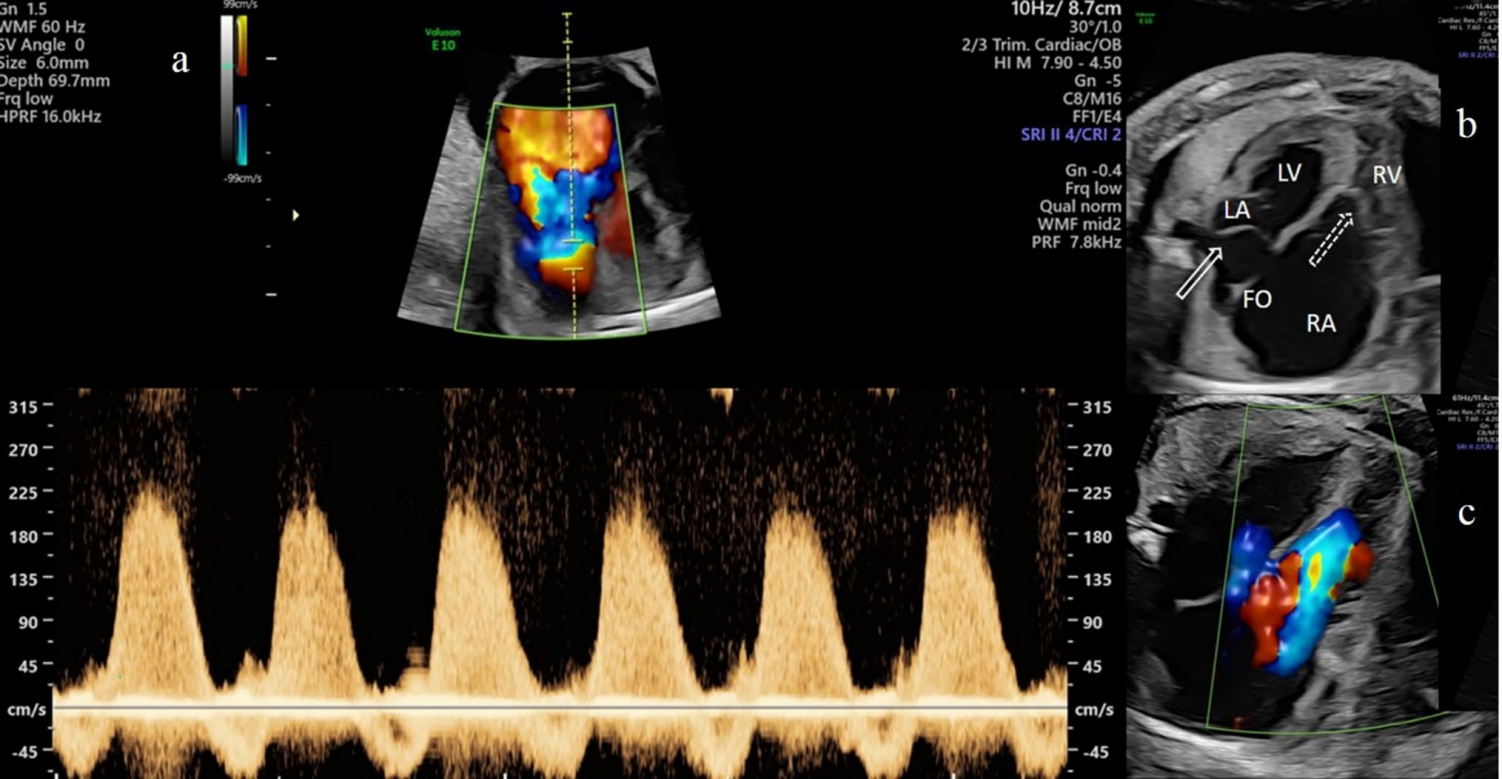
Case 1: a Holosystolic tricuspid regurgitation with peak velocity of nearly 230 cm/s shown in a fetus with severe Ebstein anomaly at 31 + 6 weeks of gestation. Color contours appears as a “hot air balloon”. b Apical four chamber view in grey scale demonstrates the severe cardiomegaly with the typical apical displacement of the tricuspid valve (open dotted arrow), with a FO (foramen ovale) flap nearly filling the entire LA (left atrium) (open arrows). The RA (right atrium) is dilated including functionally atrialized part of the right ventricle (Celermajer index: 3.23). c Color Doppler shows the anatomic origin of the regurgitant jet arising deep in the anatomical RV
